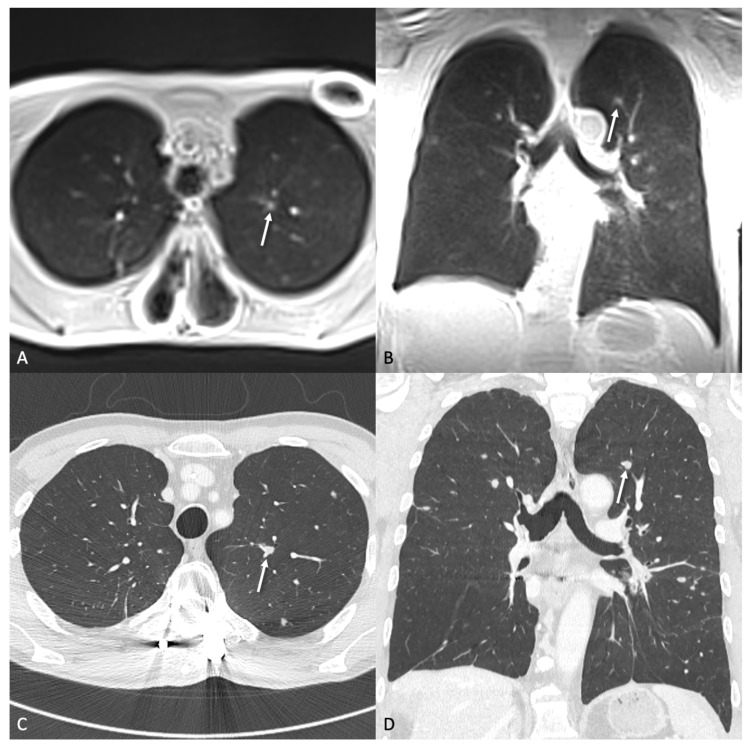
A nodule (arrow) with a size of 6.1 × 4.1 mm in the left upper lobe in a 61-year-old patient with colorectal carcinoma. The nodule was not detected in the blinded analysis of the MRI by the reader probably because of the adjacent lung vessels. In direct comparison with CT, it is possible to clearly identify the nodule in transversal (A) and coronal (B) ultra-short echo time (UTE) MRI compared to respective CT images (C,D).
